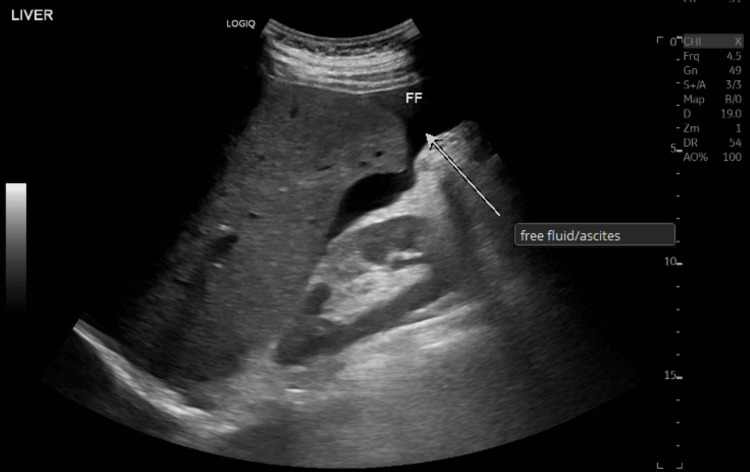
An ultrasound hepatic vein doppler revealed an accumulation of free fluid (indicated by the white arrow)
